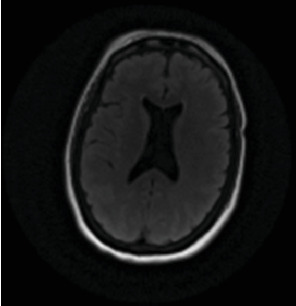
MRI performed upon admission revealed no abnormalities.
